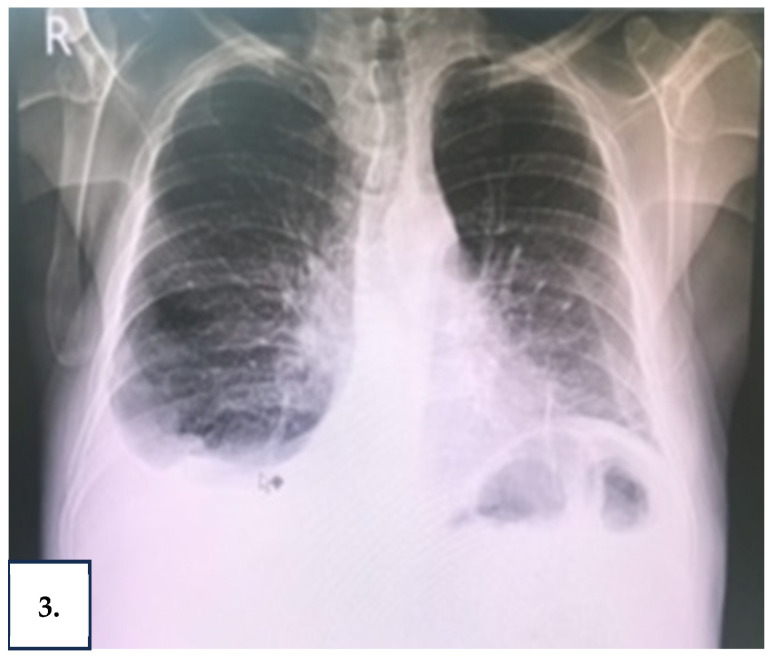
Chest radiograph showing a large right-sided pleural effusion with ipsilateral basal atelectasis, a common pulmonary manifestation of YNS.
